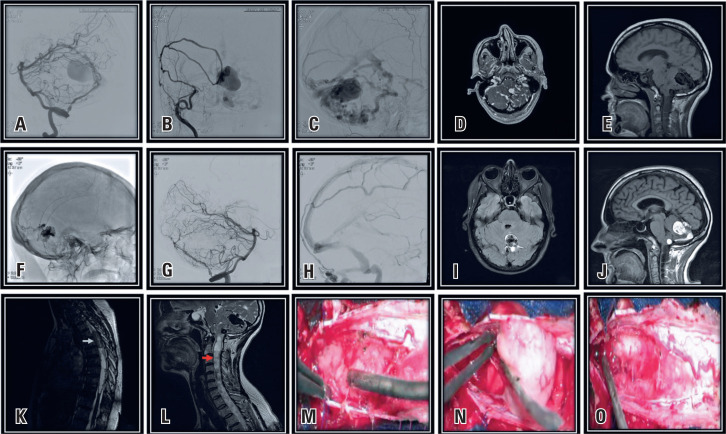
A-C) Cerebral angiography showing the presence of high-flow dural arteriovenous fistula (DAVF), along with venous aneurysm, being nourished by the superficial temporal and occipital arteries and middle pharyngeal branches; D-E) Cerebral MRI showing DAVF in posterior fossa; F-H) Cerebral angiography after endovascular treatment of DAVF; I-J) MRI after endovascular treatment with Onyx; K) Thoracic spine MRI showing intramedullary tumor; L) MRI of cervical spine showing syringomyelia extending from medullary tumor to medulla; M-O) Transoperative images of spinal tumor resection
